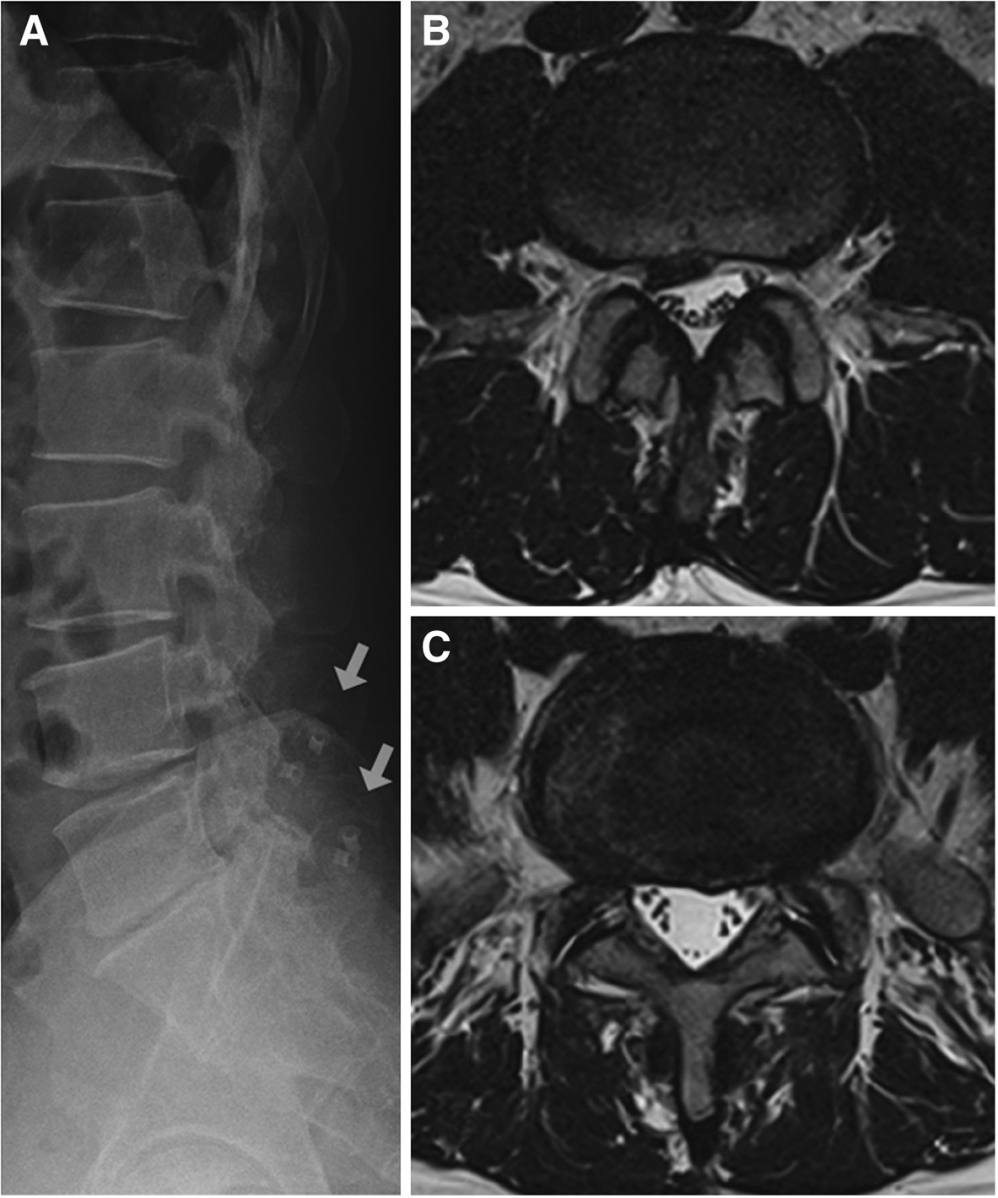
Lumbar imaging studies. (A) Simple lateral radiographs with the DIAM^®^ interspinous device (white arrows). (B) Focal disc protrusion at L4/5. (C) Diffuse protrusion at L5/S1.
